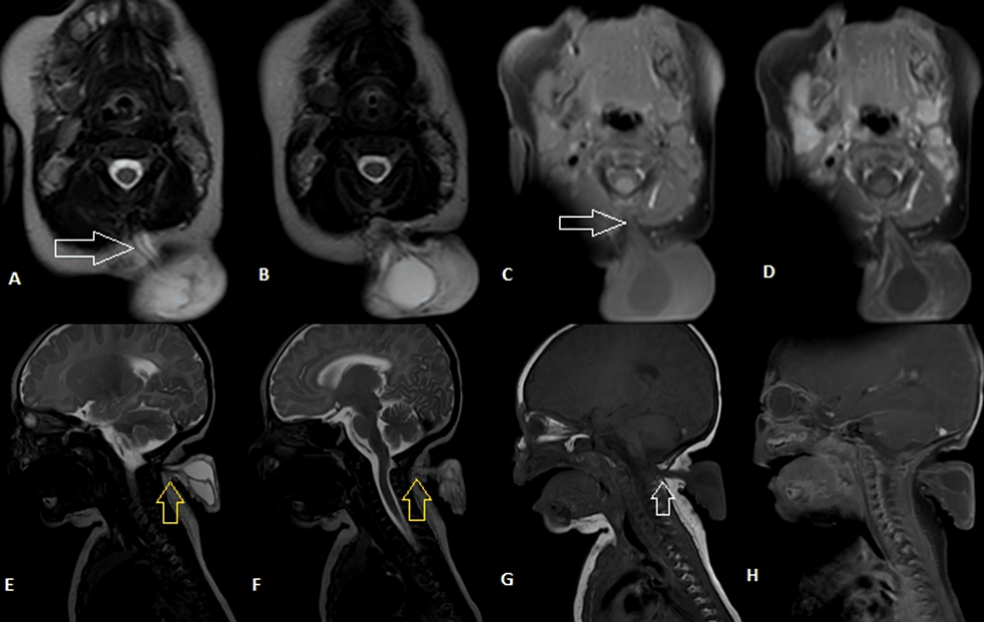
Cranial and cervical MRI of the patient after arrival at the outpatient clinic. A, B: axial T2-weighted images; C, D: axial T1-weighted images without and with gadolinium, respectively. The white arrow points to the connection between the cervical mass and the spinal cord; E, F: coronal T2-weighted images; G, H: coronal T1-weighted images without and with gadolinium, respectively. Yellow arrows point to the dermal connection of the mass. Image courtesy: Radiology Department of Al Rasoul Al Aazam Hospital
